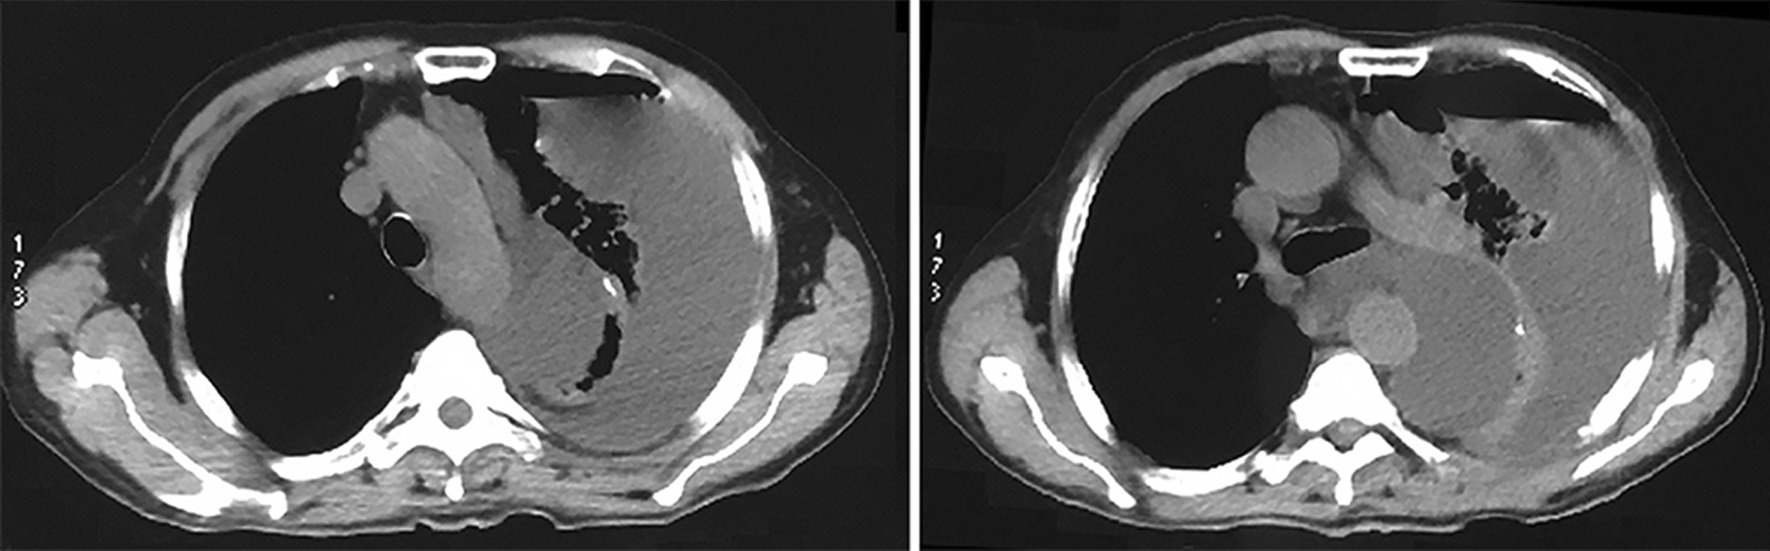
The thoracic CT scan showed a huge cyst full of fluid in the left thoracic cavity
